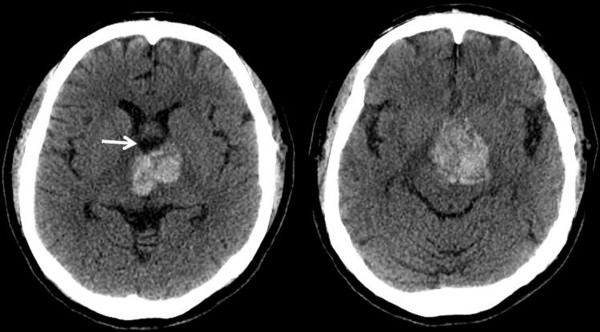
Axial non-contrast CT images show a large and heterogeneously hyperattenuated hemorrhagic mass in the suprasellar area, with dilatation of the anterior part of the third ventricle (arrow).
